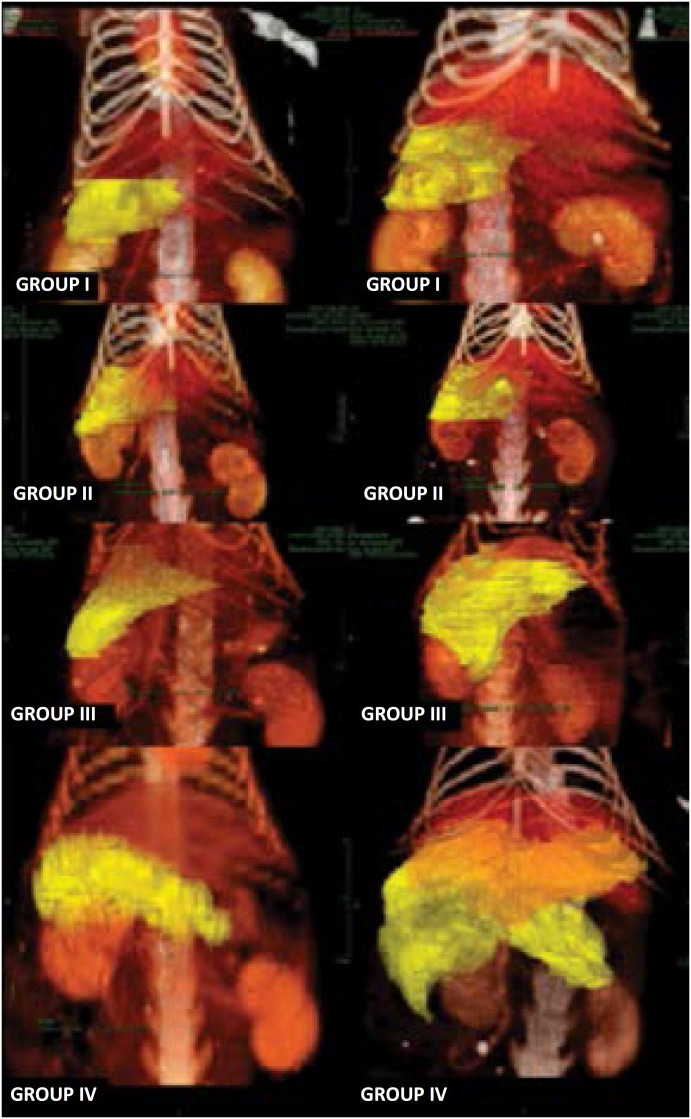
3D CT scan with volumetric reconstruction. Before
